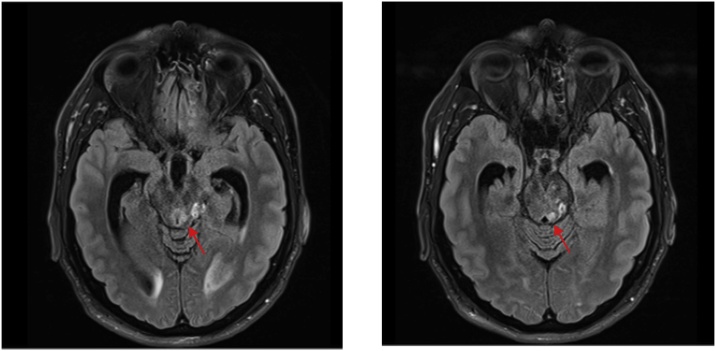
MRI brain axial FLAIR sequences showing tumour extension along left periaqueduct (arrows).
